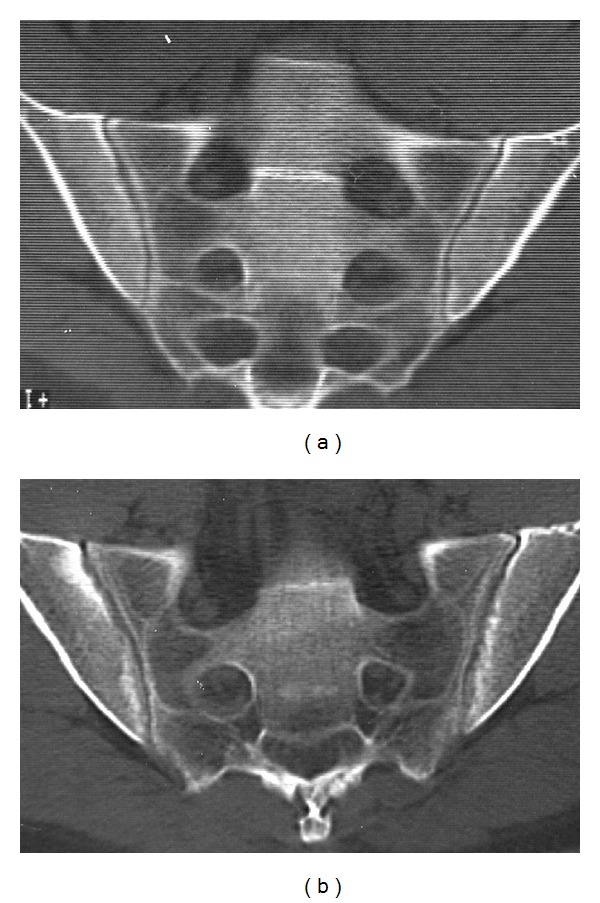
A 37-year-old male with 1–3 years duration of inflammatory low back pain. (a) The initial CT shows unilateral sacroiliitis with subtle but definite erosions on the iliac side of the right sacroiliac joint. (b) After 5.5 years, there is progression to bilateral moderately advanced sacroiliitis.
